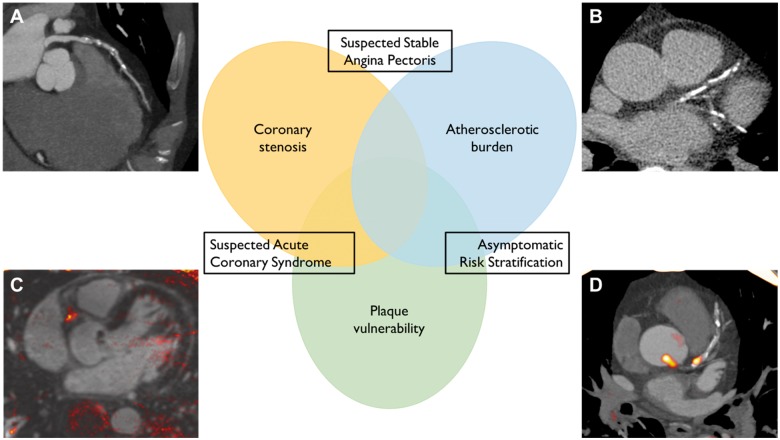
Complementary roles of non-invasive coronary imaging. The use of non-invasive coronary imaging can be considered in three contexts, each with specific objectives that may inform the choice of imaging modality. (*A*) Computed tomography angiography provides accurate assessment of coronary stenosis that can guide management of patients with suspected stable angina and rule out Type 1 myocardial infarction in patients with potential acute coronary syndrome. (*B*) Coronary artery calcium scanning is able to reliably quantify overall atherosclerotic burden and improve risk stratification in asymptomatic individuals. (*C*) T_1_-weighted magnetic resonance coronary angiography can identify features of atherosclerotic instability including intracoronary thrombus and intraplaque haemorrhage and may be of value in suspected acute coronary syndrome or asymptomatic risk stratification. (*D*) Positron emission tomography can employ specific tracers designed to target markers of plaque vulnerability that may improve prognostic assessment or act as a surrogate of therapeutic response in asymptomatic patients.
